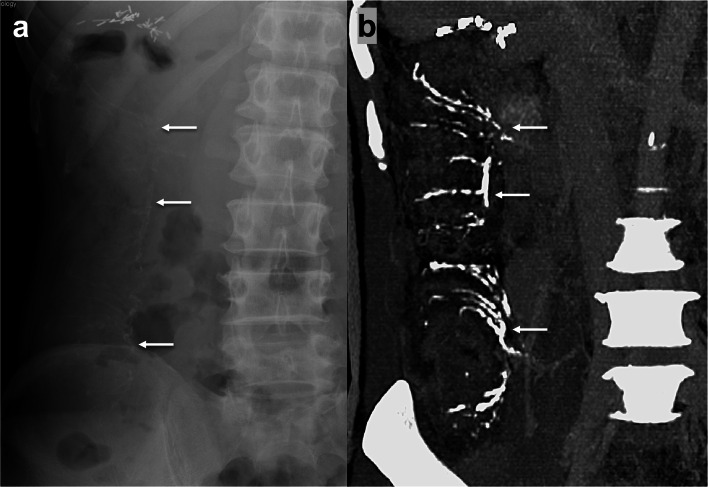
a Curvilinear calcifications alongside the right colon seen on abdominal radiograph. b Corresponding maximum intensity projection display of CT angiography shows comprehensive demonstration of the calcified straight and marginal veins of the ascending colon
